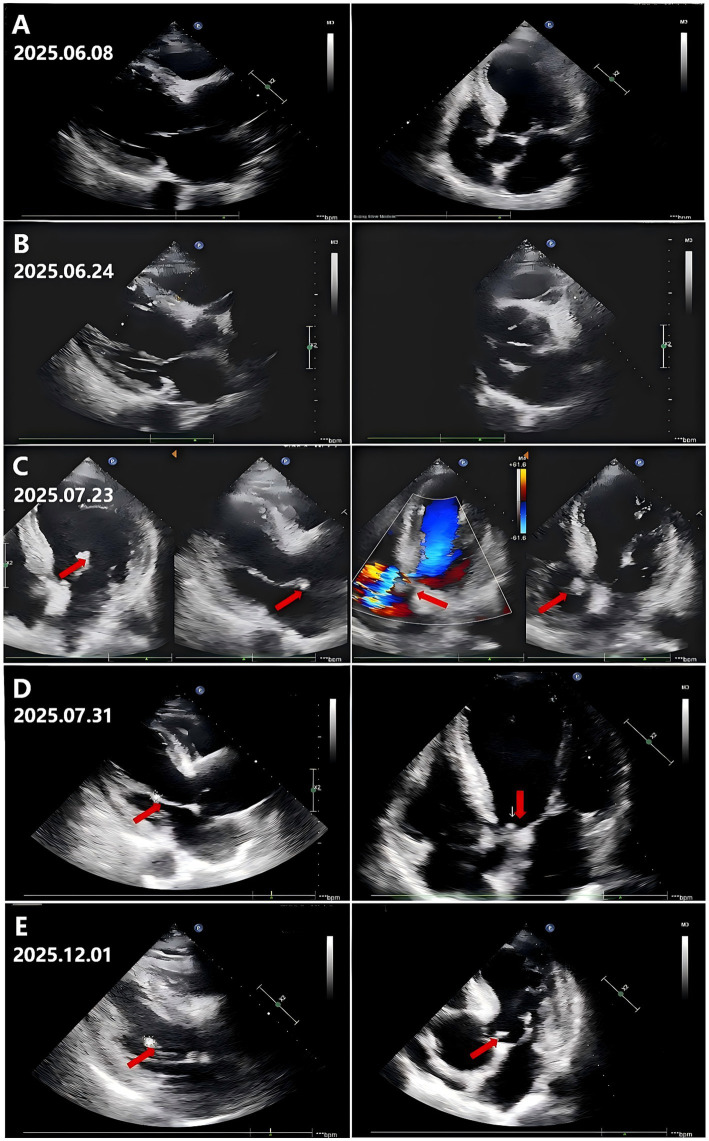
The transthoracic echocardiography results of the mitral and aortic valves. (A) No vegetations in the mitral valve (left ventricular long-axis view) and aortic valve (five-chamber view) on 5th day. (B) No vegetations in the mitral valve (left ventricular long-axis view) and aortic valve (short-axis view of the aorta) on 20th day. (C) Mitral valve vegetation 11 × 8 mm (left ventricular long-axis and four-chamber views) and aortic valve 18 × 15 mm (five-chamber view) vegetation on 49th day. (D) Mitral valve vegetation 10 × 7 mm (left ventricular long-axis view) and aortic valve 6 × 4 mm (five-chamber view) vegetation on 56th day. (E) Mitral valve vegetation 10 × 7 mm (left ventricular long-axis view) and aortic valve 7 × 5 mm (five-chamber view) vegetations on 86th day. Red arrows indicate the location of the vegetations.
